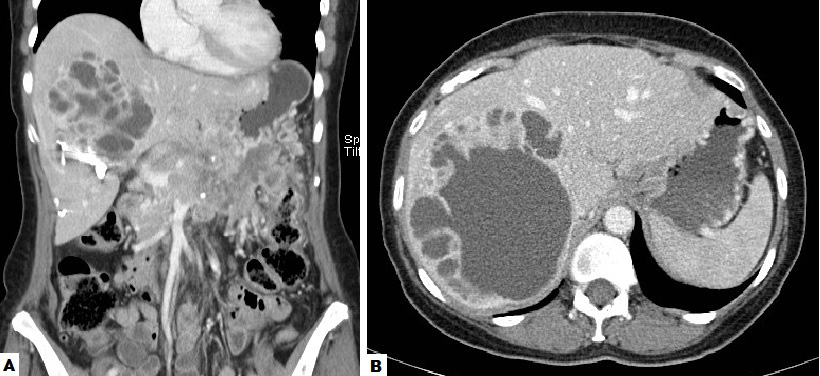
a: CT demonstrating pancreatic mass with superior mesenteric/portal vein encasement and associated liver metastasis (Case 2); b: CT demonstrating right liver metastasis (post embolization; Case 2).
